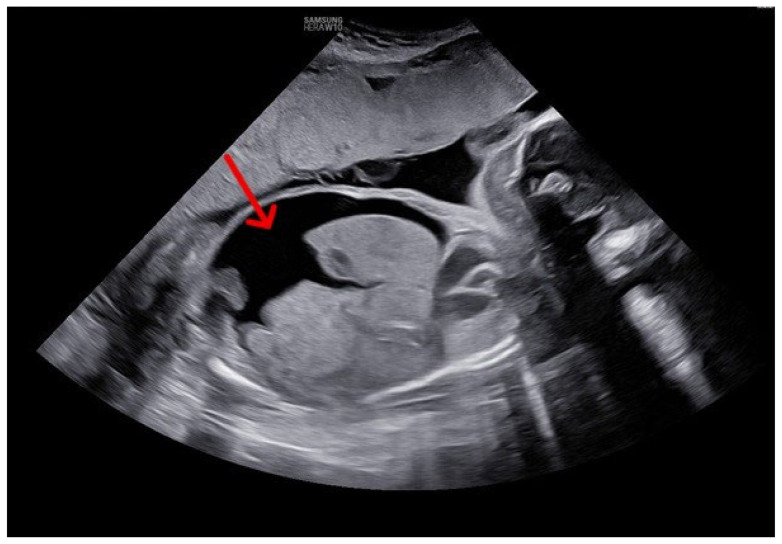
Ultrasound image obtained at the time of hospital admission and diagnosis, demonstrating massive fetal ascites (red arrow) at 24 weeks of gestation. Sagittal view of the fetal abdomen.
